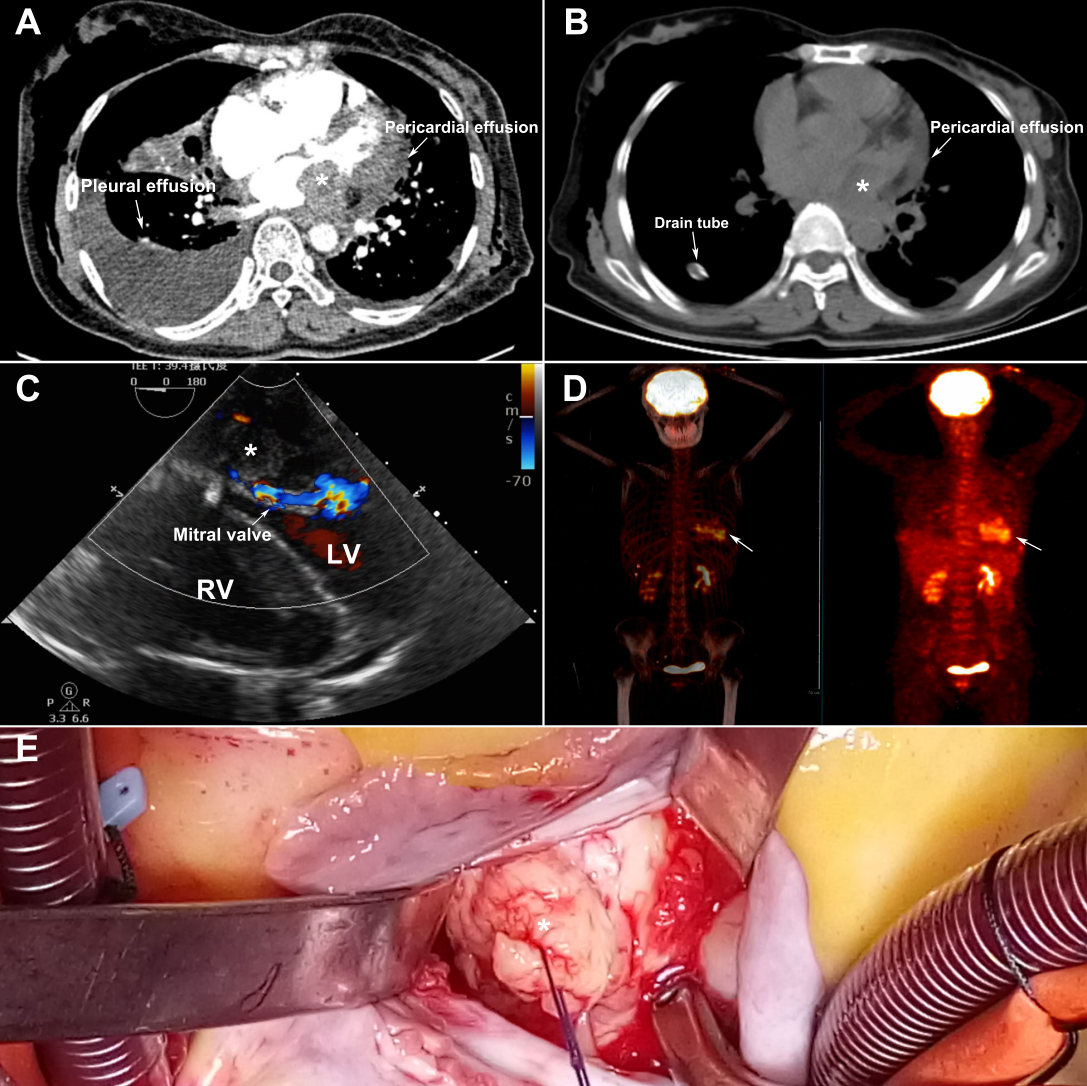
Preoperative examinations and intraoperative findings: a CT scans revealed occupying lesion in left atrium and massive hydropericardium and pleural effusion (asterisk and arrows); b The massive right pleural effusion reduced significantly after chest drainage; c TEE showed the left atrial mass limited the mitral valve inflow significantly; d PET/CT confirmed high grade fluorodeoxyglucose uptake only in the intracavitary mass (arrows); e Operative exploration confirmed the atrial mass had invaded the mitral annulus and posterior wall of left ventricle. * The left atrial mass; RV, right ventricle; LV, left ventricle; TEE, Transesophageal echocardiography; PET/CT, Positron emission tomography/computed tomography
